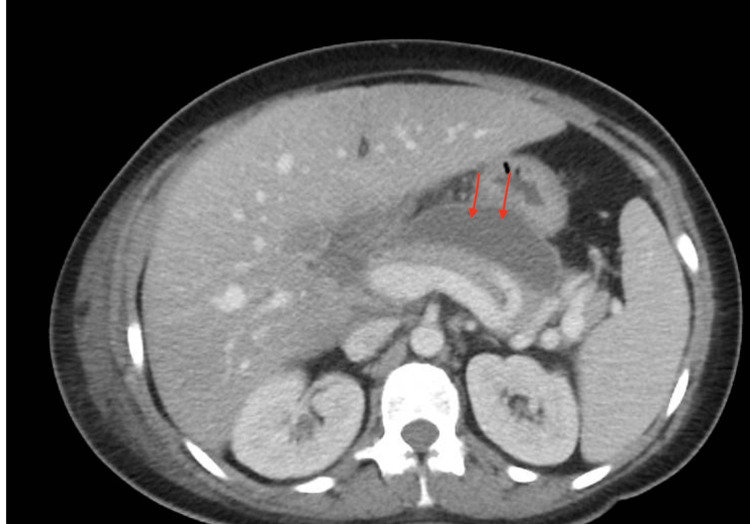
CT abdomen and pelvis noting findings of a collection within the pancreatic less sac measuring approximately 8.3 x 2.5 cm transaxially, suggestive of a pancreatic pseudocyst. CT: computed tomography.
